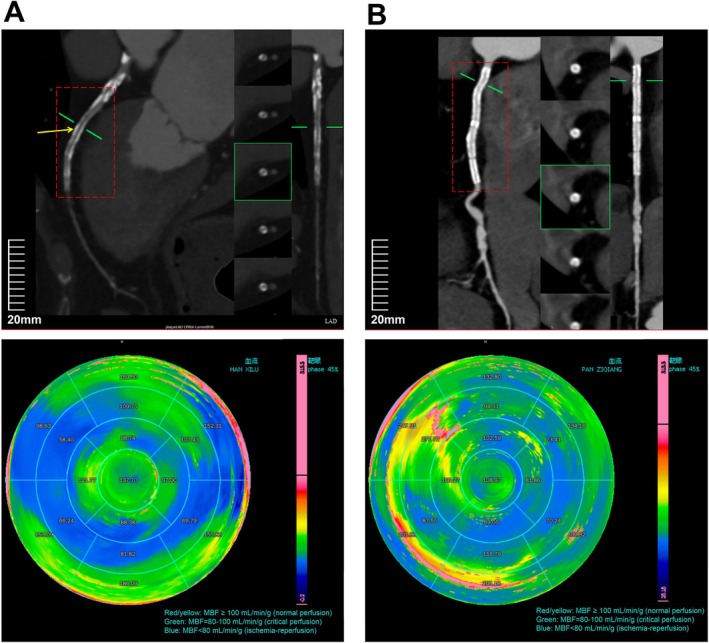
Comparison of CT imaging characteristics in patients with ISR. (A) ISR^+^ patient: (top) coronary CTA curved planar reformation image showing severe ISR in the left anterior descending stent (yellow arrows, CTA% DS = 72%), measured by non‐invasive (APEX‐CT CTA); (bottom) CT‐MPI pseudo‐color image showing myocardial perfusion defect in the anterior wall (blue area, MBF = 68 mL/min/100 g). (B) ISR^−^ patient: (top) coronary CTA curved planar reformation image showing stent patency (CTA% DS = 28%) and (bottom) CT‐MPI pseudo‐color image showing homogeneous normal myocardial perfusion (red area, MBF = 98 mL/min/100 g). Green arrow indicates in‐stent stenosis site. Red dotted line indicates stent location. Pseudo‐color image color scale, Red/yellow: MBF ≥ 100 mL/min/g (normal perfusion); Green: MBF = 80–100 mL/min/g (critical perfusion); Blue: MBF < 80 mL/min/g (ischemia–reperfusion).
